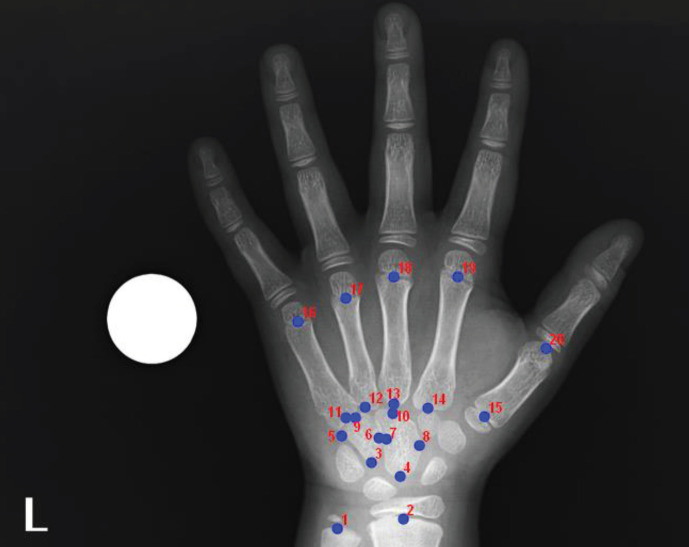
Landmarks (1-20) on the left-hand radiography used in this study were shown.
